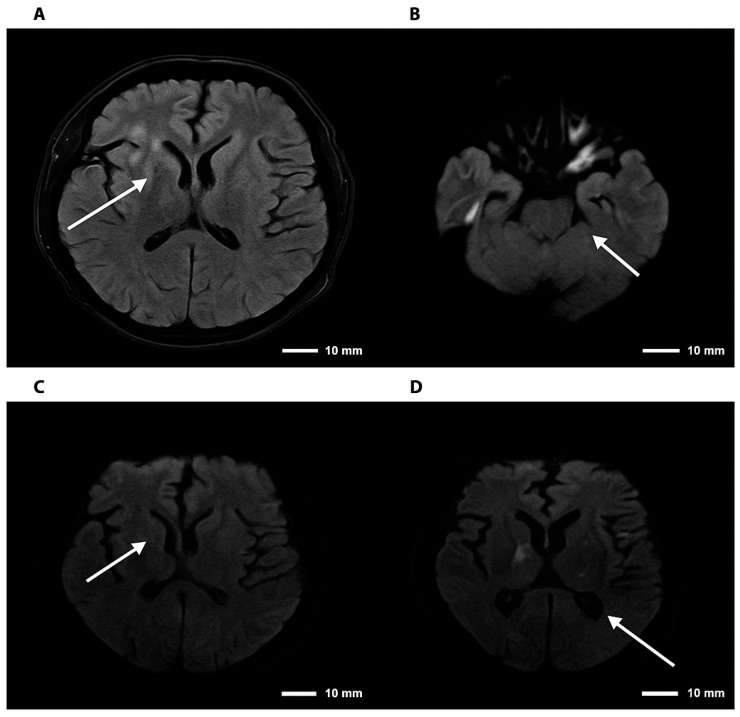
Brain magnetic resonance imaging—FLAIR and DWI sequences in cryptococcal meningoencephalitis. Note. Brain magnetic resonance imaging. (A) Axial FLAIR sequence showing hyperintensity in the right basal ganglia. (B) DWI sequence demonstrating diffusion restriction in the right thalamic region. (C,D) Axial DWI images confirming foci of restriction in the right basal ganglia and thalamus, consistent with an infectious/inflammatory process. The arrows indicate lesions consistent with cryptococcomas, involving the supra- and infratentorial parenchyma to varying degrees.
